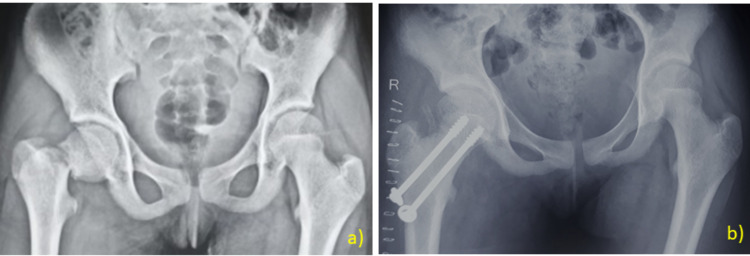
Case Example: Open Reduction and Fixation With Screws a) A 14-year-old female patient with Delbet type II fracture presented 12 hours after the injury. The patient was taken to the operating room and closed reduction was unsuccessful. Open reduction through the anterolateral approach and fixation with cannulated screws was done. b) Postoperative radiographs.
